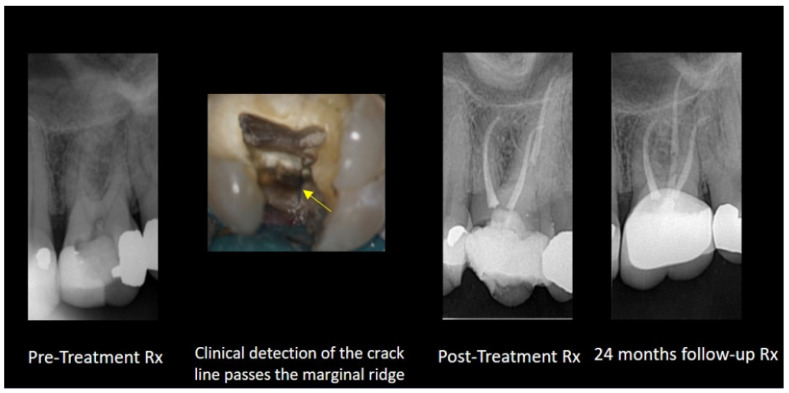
Maxillary first molar with a crack (yellow arrow demonstrates the crack line as the cavity is accessed). The tooth is obturated with the single-cone technique with GP and ERS. X-ray images of pre- and post-treatments and from a two-year follow up.
